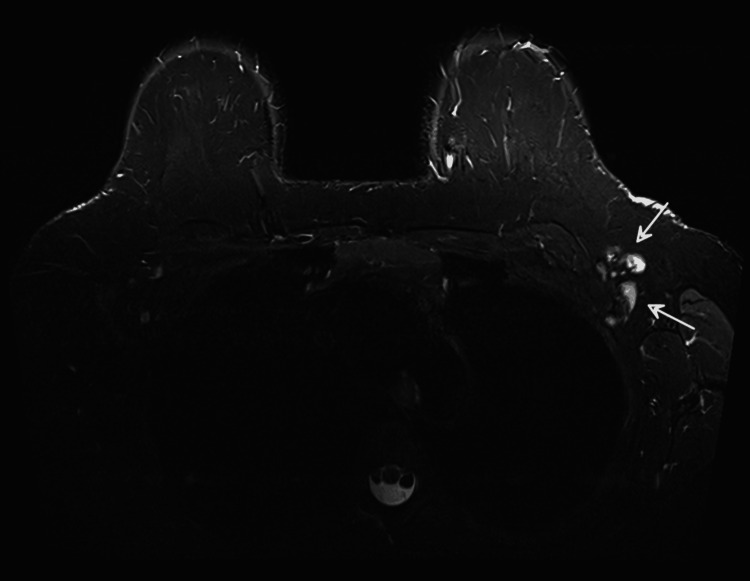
T2-weighted STIR breast MRI Axillary lymph nodes with cortical thickness up to 7 mm (white arrows). STIR: short tau inversion recovery; MRI: magnetic resonance imaging
